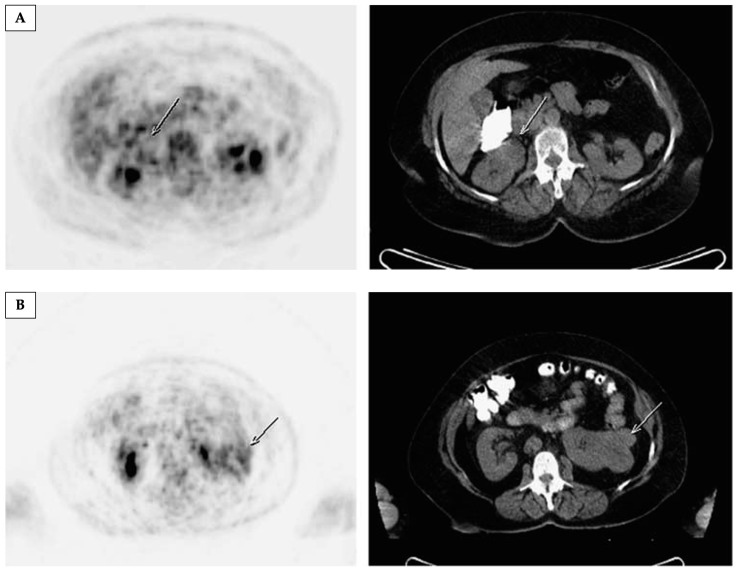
[^18^F]FDG-PET cannot accurately differentiate between malignant and benign renal masses. (A) Axial PET and CT images from a [^18^F]FDG-PET/CT study showing low-grade tracer uptake in a right renal mass (arrowed), which was later confirmed to be renal cell cancer. (B) Axial slices of PET and CT from a second patient showing some [^18^F]FDG uptake in a left renal mass (arrowed), which was later shown to be an oncocytoma. Reprinted, under a CC BY license (license number: 5962670886654) as mediated by the Copyright Clearance Center (Chicago, IL, USA), from reference [93].
